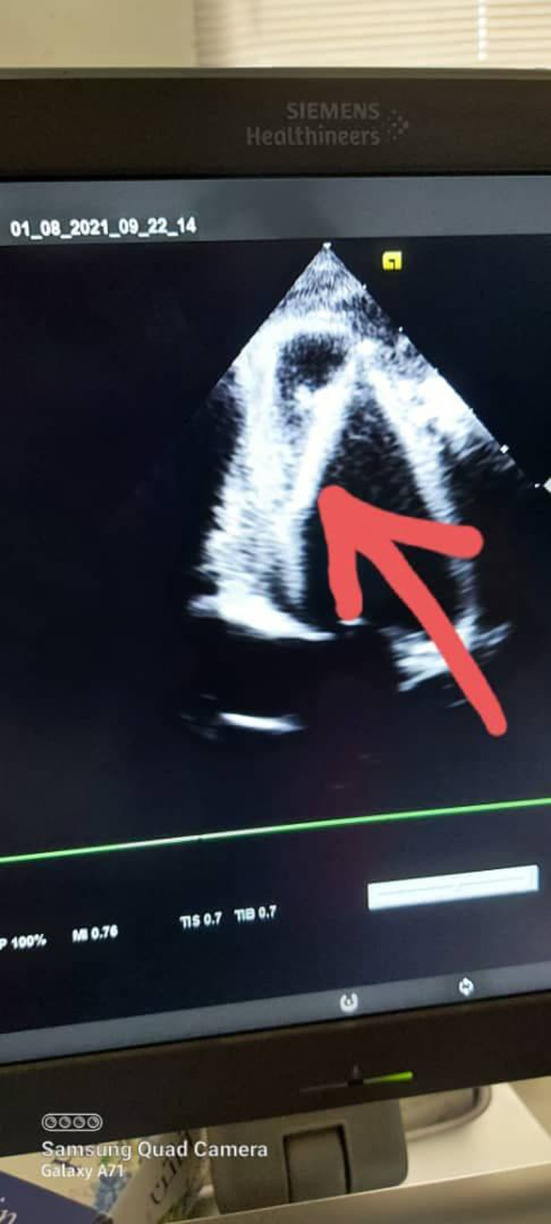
Echocardiography showing a thorn (arrow) within the left ventricle that is penetrating from the apex posteriorly
